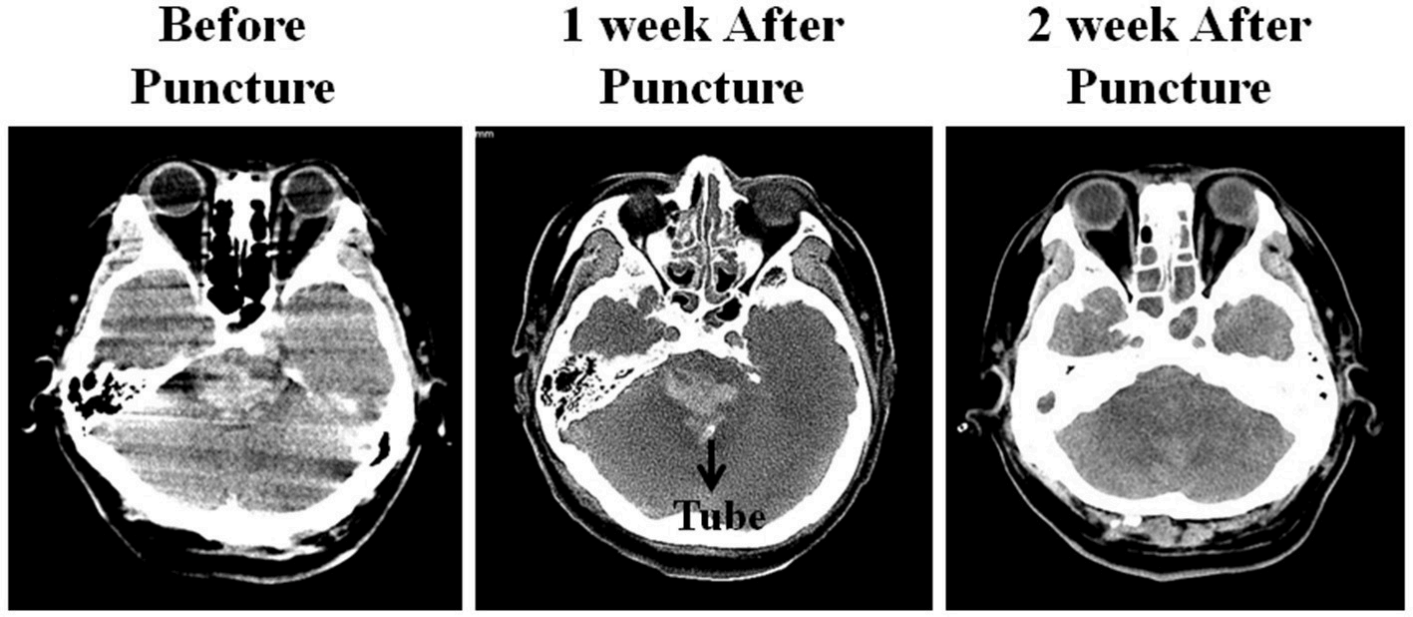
The hematoma condition showed on Head CT examination: (A) Before puncture, (B) no significant change of the hematoma 1 week after the procedure, (C) part of the hematoma was resolved 2 weeks after the procedure.
